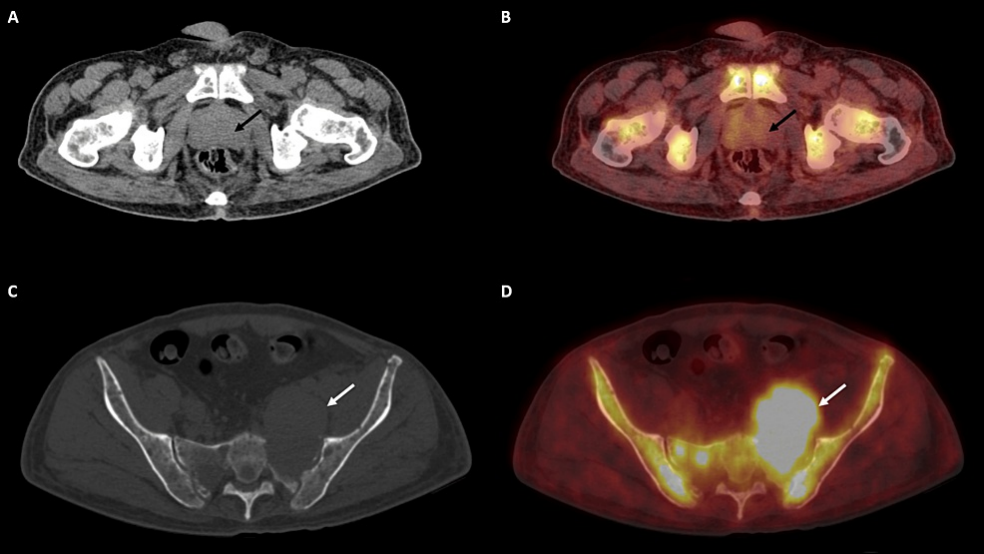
Axial CT image (A) and fused PET CT image (B) reveal an enlarged prostate gland (black arrows) with no abnormal FDG avid lesion. Axial CT image (C) and fused PET CT image (D) reveal a lytic lesion involving the left ala of the sacrum with FDG avid soft tissue component (white arrows). Other FDG avid lytic lesions are also noted in pelvic bones and sacrum. FDG: fluorodeoxyglucose; CT: computerized tomography.
